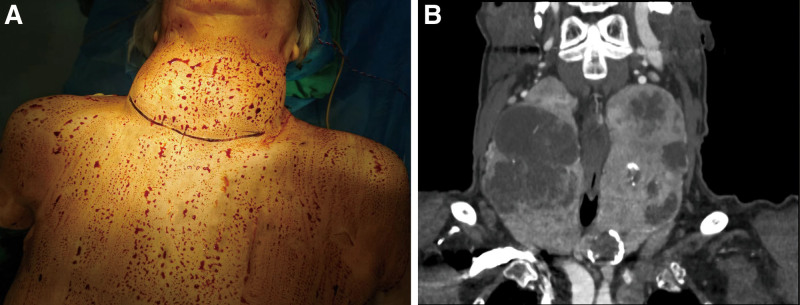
The preoperative findings. (A) Inspection: thy thyroid gland enlarged diffusely; (B) Coronal section of computed tomography showed diffuse enlargement of the 2 lobes of the gland.
